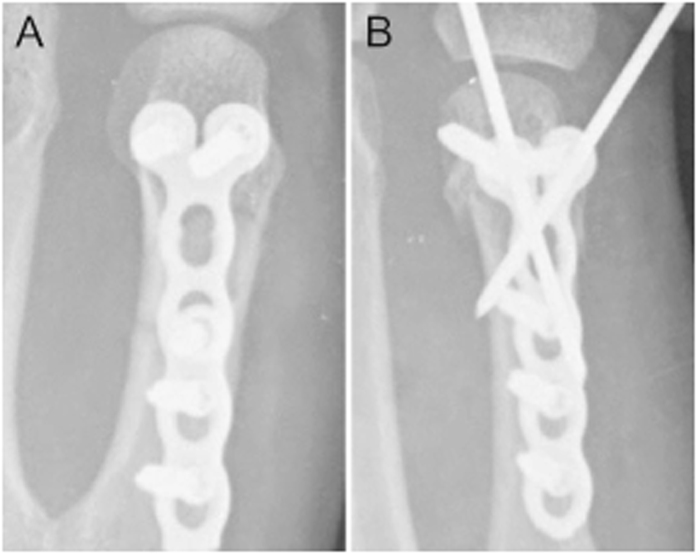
Representative postoperative radiographs of (A) locking plate alone and (B) locking plate in combination with two K-wires on antero-posterior view.
